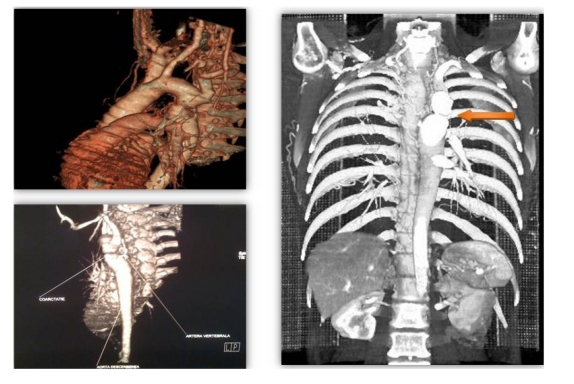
CT 3D images showing significant coarctation of the thoracic aorta beyond the origin of the left subclavian artery (arrow) with poststenotic dilatation and hypoplastic abdominal aorta
